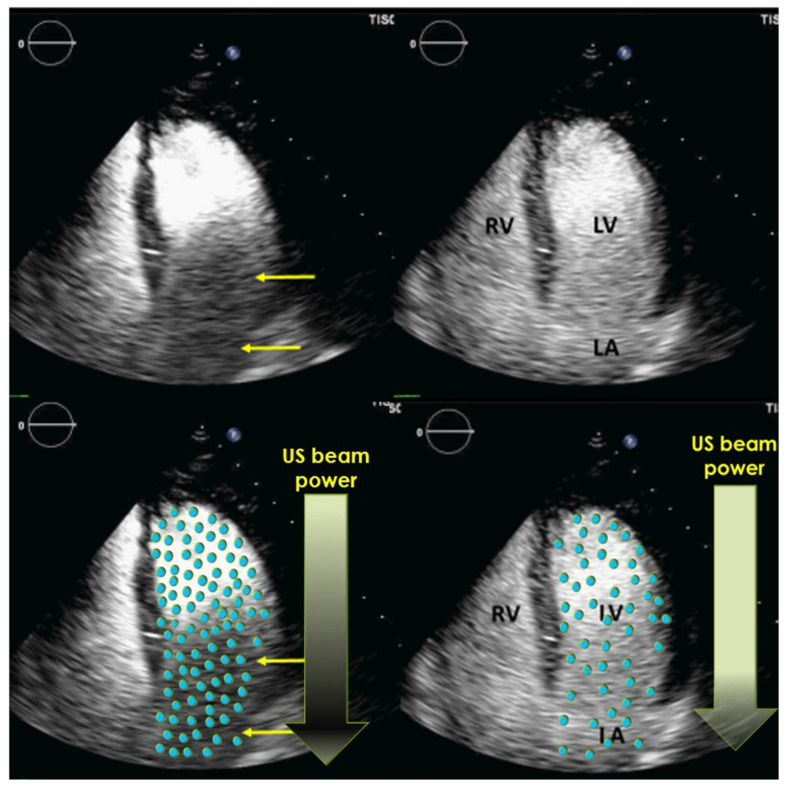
Contrast shadowing: 4-chamber view (left) due to high concentration of microbubbles in the LV early after bolus injection. The ultrasound is attenuated in the nearfield and does not reach the basal LV cavity. At greater depth the microbubbles do not resonate which causes poor LV opacification (yellow arrows). Notably, 20 s later and without any change in machine settings (right), there is a higher degree of dilution of the microbubbles, and there is also enough power from the ultrasound to resonate the microbubbles at a greater distance from the transducer. US: ultrasound.
